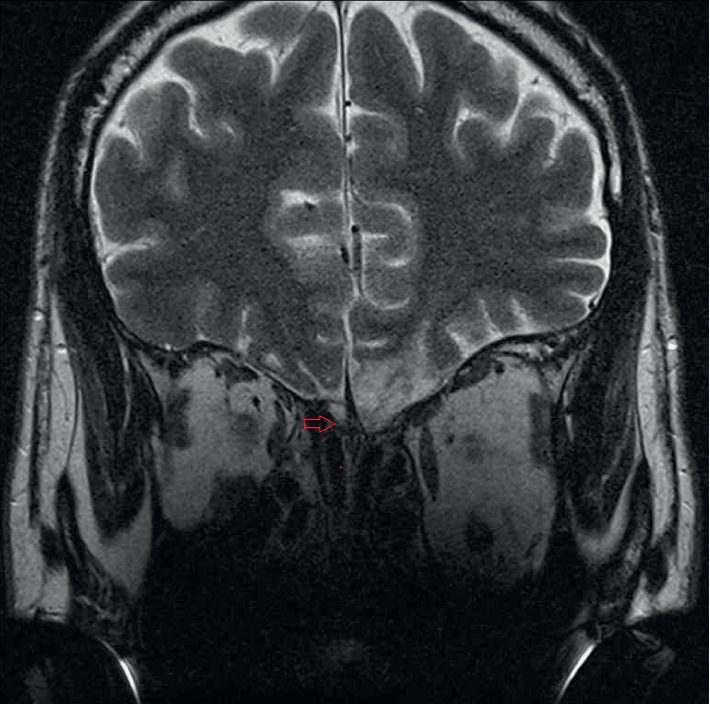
MRI shows irregular hyperintensity over bilateral rectus gyri, with extensive tissue loss at the left frontal base. The sizes of the right olfactory bulb (red arrow) and tract were relatively small.
